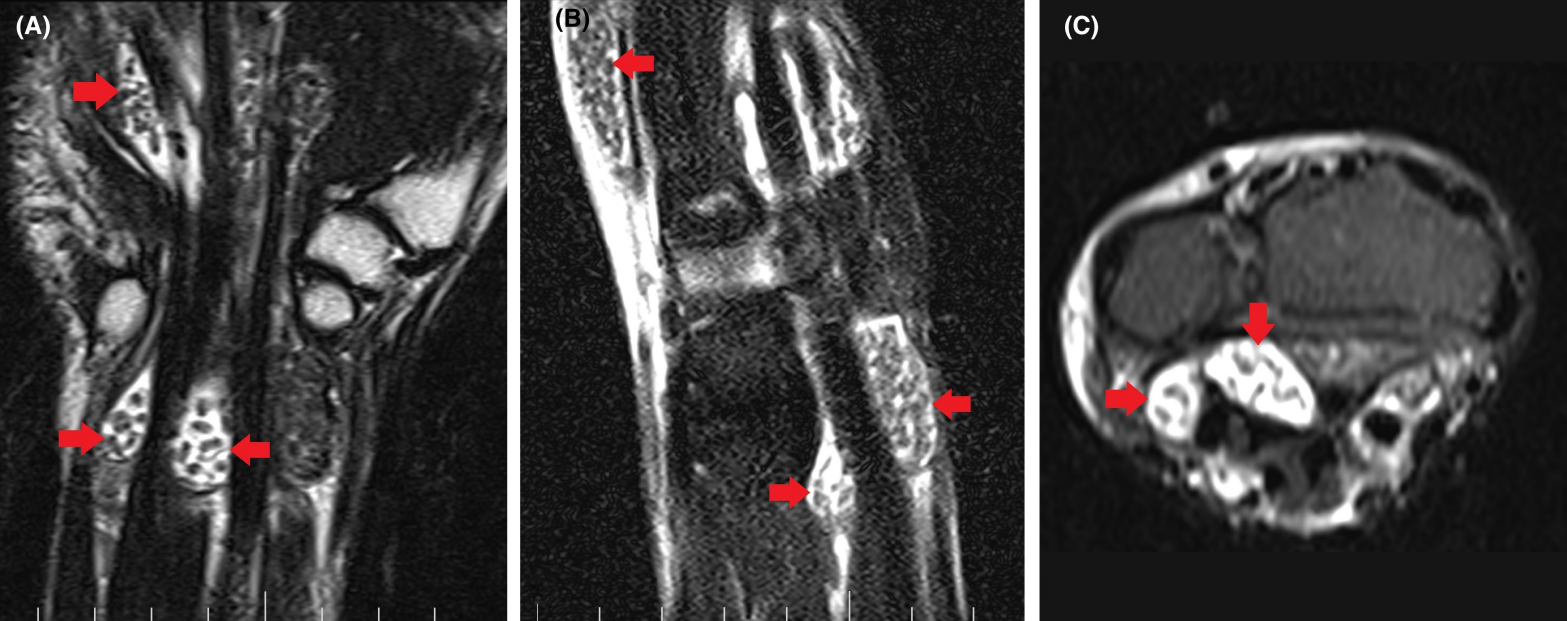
T2 sequences of the left wrist MRI; (A) coronal view, (B) sagittal view, (C) axial view.
